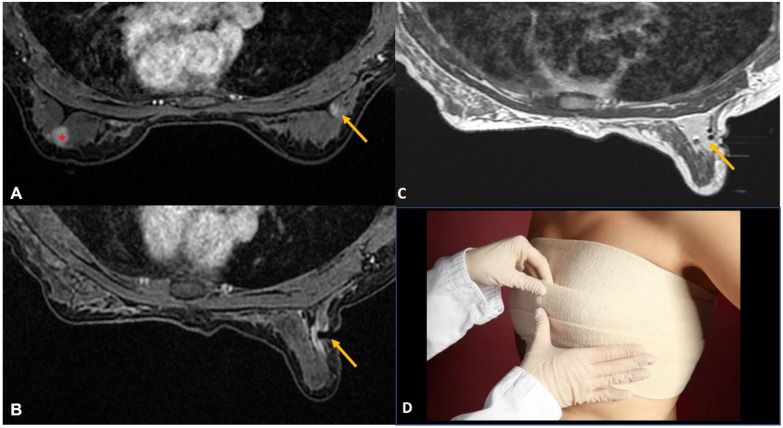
(A). Staging MRI in a woman with known left breast cancer (red asterisk) showed focal non-mass enhancement in the right breast (yellow arrow). (B). MRI-guided biopsy to rule out contralateral synchronous cancer showed the tip of the obturator at the right location (yellow arrow). (C). Post-biopsy non-fat saturated image showed a large hematoma at the biopsy site almost occupying the upper outer quadrant (yellow arrow). (D). Tight compression bandage applied to the breast after the biopsy reduces the risk of further bleeding significantly.
